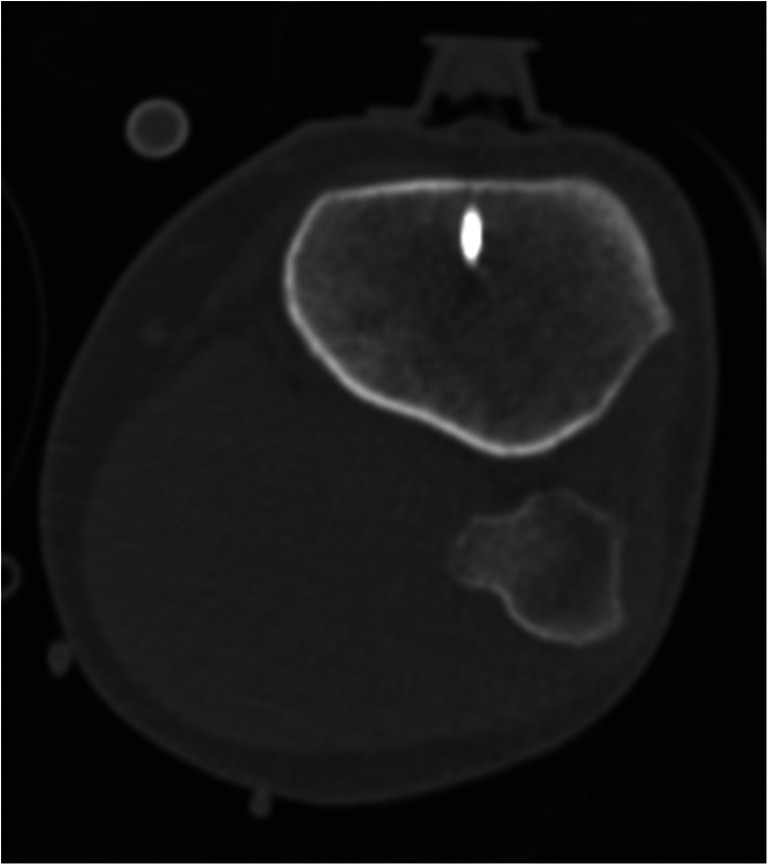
Short low-dose CT scan over the IO device. Exemplary, short CT scan over the IO device prior to the main examination to confirm correct placement within the intramedullary cavity (other patient)
